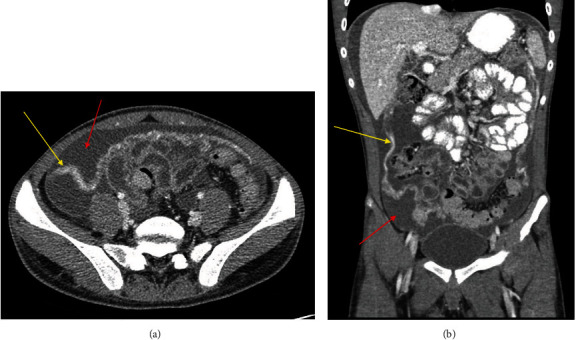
Contrast-enhanced axial (a) and coronal (b) CT images demonstrating omental thickening (yellow arrows) and ascites (red arrows).
